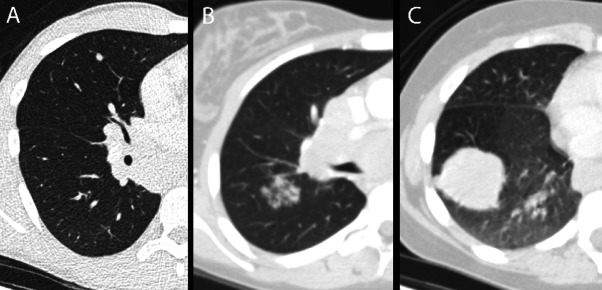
Different morphologic patterns of lung lesions in patients with pediatric classical Hodgkin lymphoma. Examples of different lesions present on initial staging CT images on axial slices at lung window settings (center: -600 HU; width: 1500 HU), that were classified according to the current recommended terms of the Fleischner Society. (A) nodules (B) consolidations (C) masses
